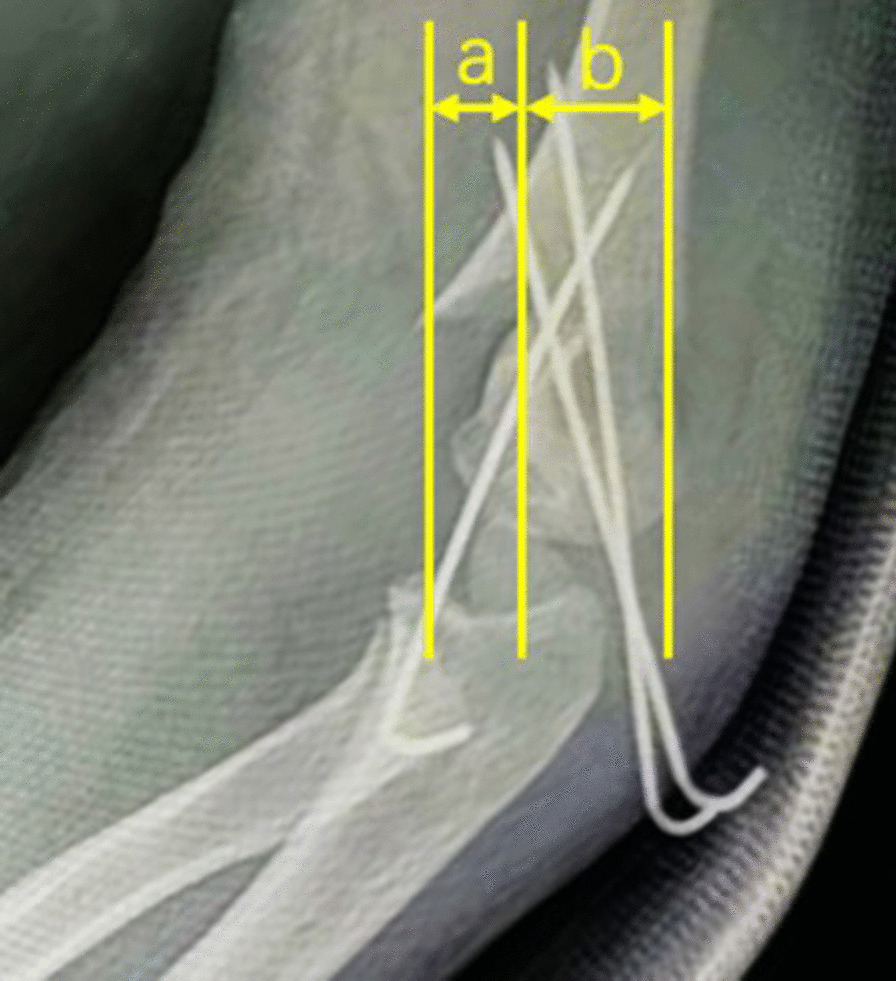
Measurement of LRP. On the lateral X-ray, the forward shift of the proximal humeral cortex a was divided by the width of the distal humerus b at the fracture site, and the value was multiplied by 100 to be the LRP
